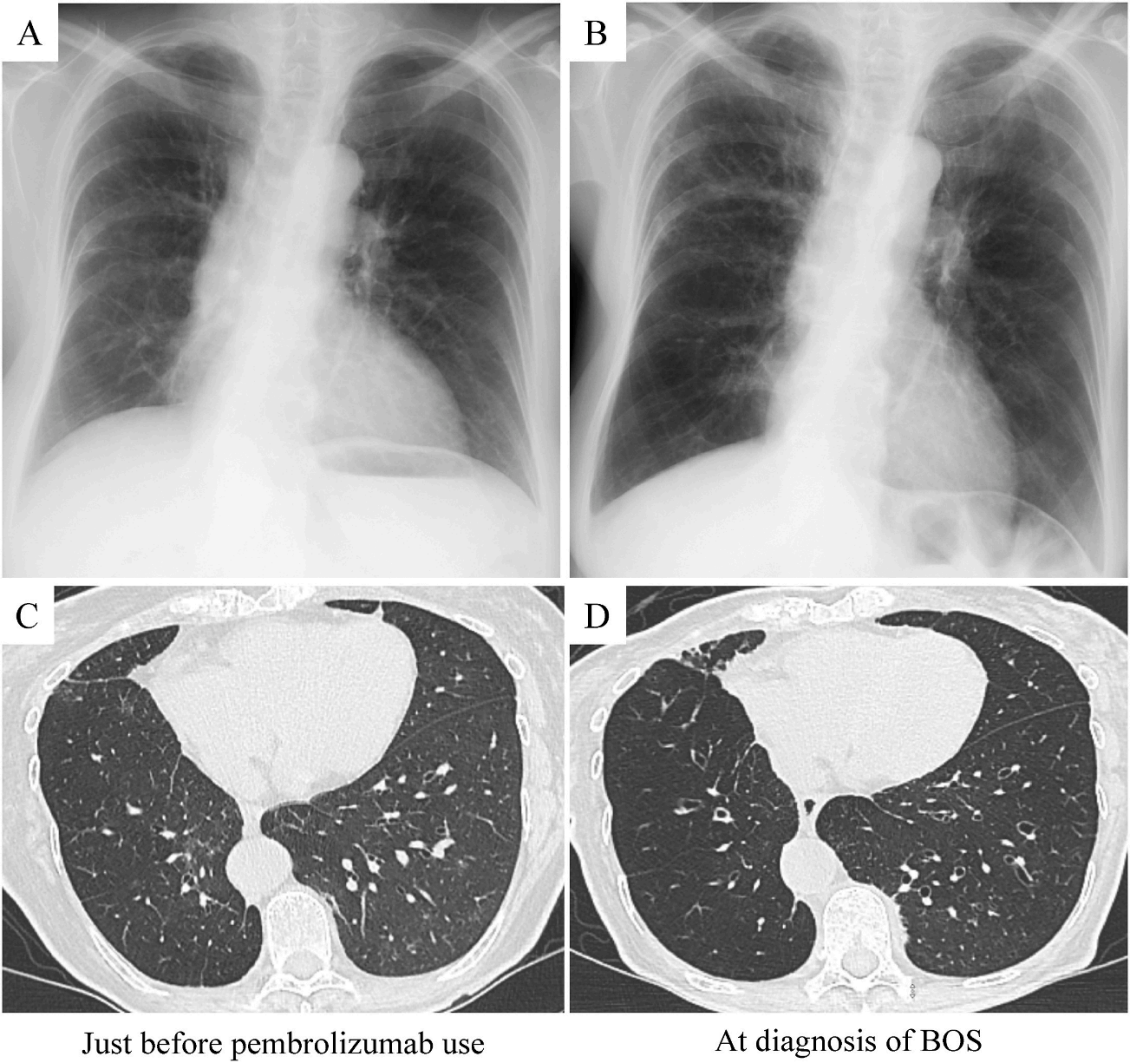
Compared to the chest radiograph results obtained at the beginning of pembrolizumab treatment (A), the radiograph results obtained at admission (B) show hyperinflation in the right lower field. Compared with the image obtained just before pembrolizumab use (C), the chest inspiratory computed tomography image at the time of the BOS diagnosis shows reduced vascular caliber and lung density in the right lower lobe and dilated airways in the both lower lobes (D).
